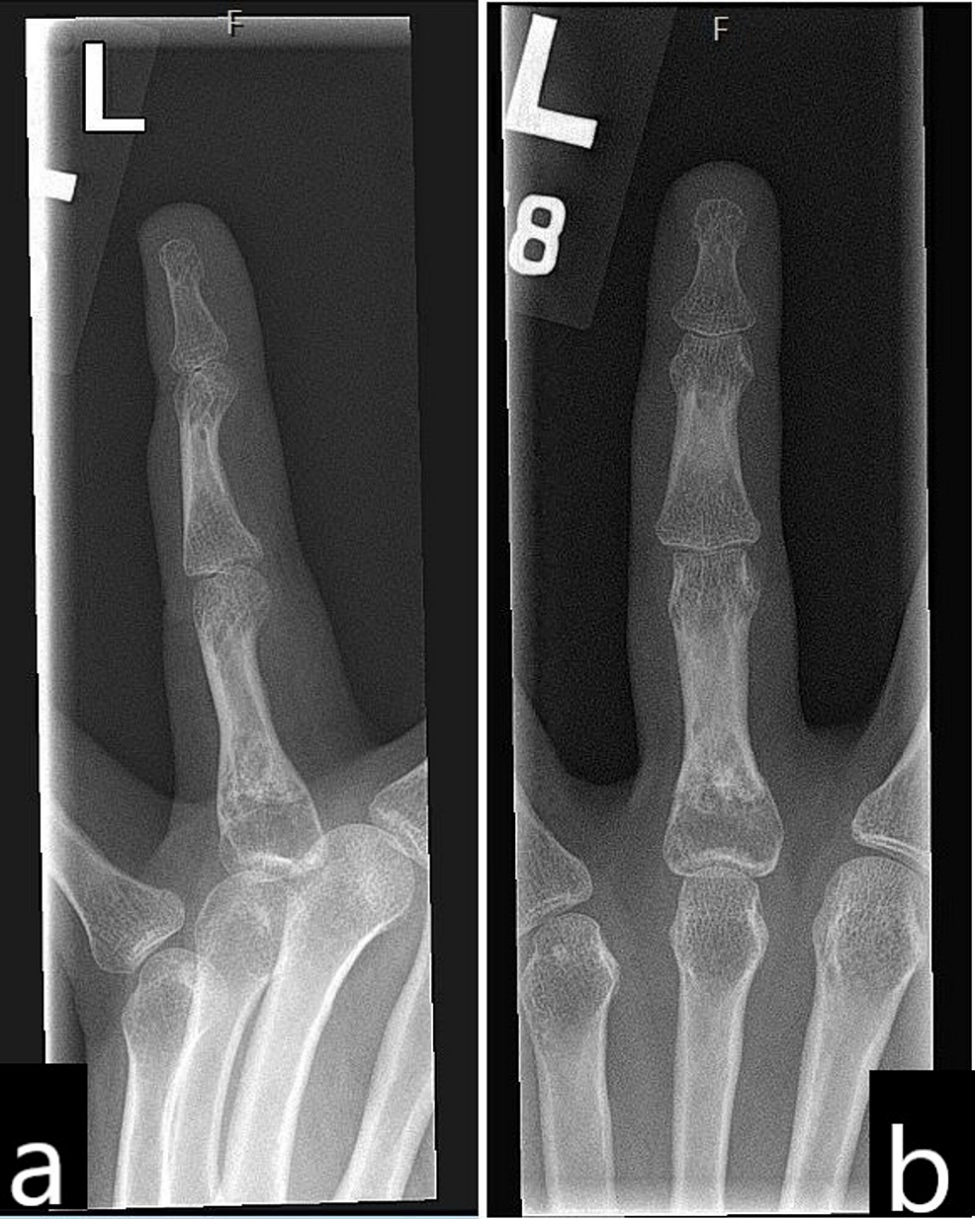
X-ray image at 15 months showing good incorporation of bone graft with no tumor recurrence.
